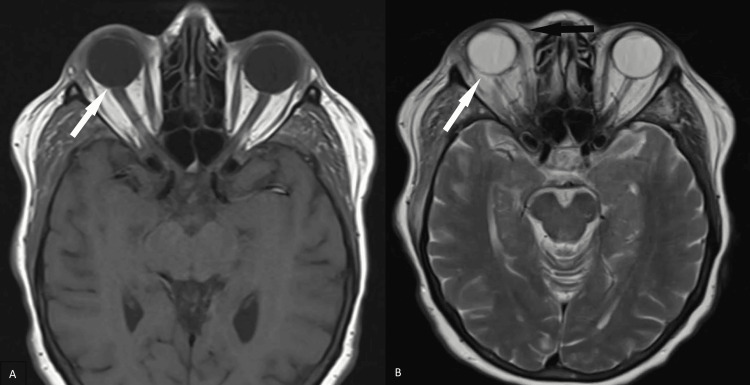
MRI images of the brain showing findings of resolved increased right intraorbital pressure and right orbital cellulitis. Axial T1-weighted and T2-weighted sequences demonstrate resolution of right posterior globe tenting (white arrows, A and B) suggestive of right intraorbital pressure normalization. The axial T2-weighted sequence also demonstrates normalization of T2 signal intensity throughout the extraconal palpebrae soft tissues overlying the epicanthal folds indicative of right orbital cellulitis resolution (black arrow, B).
